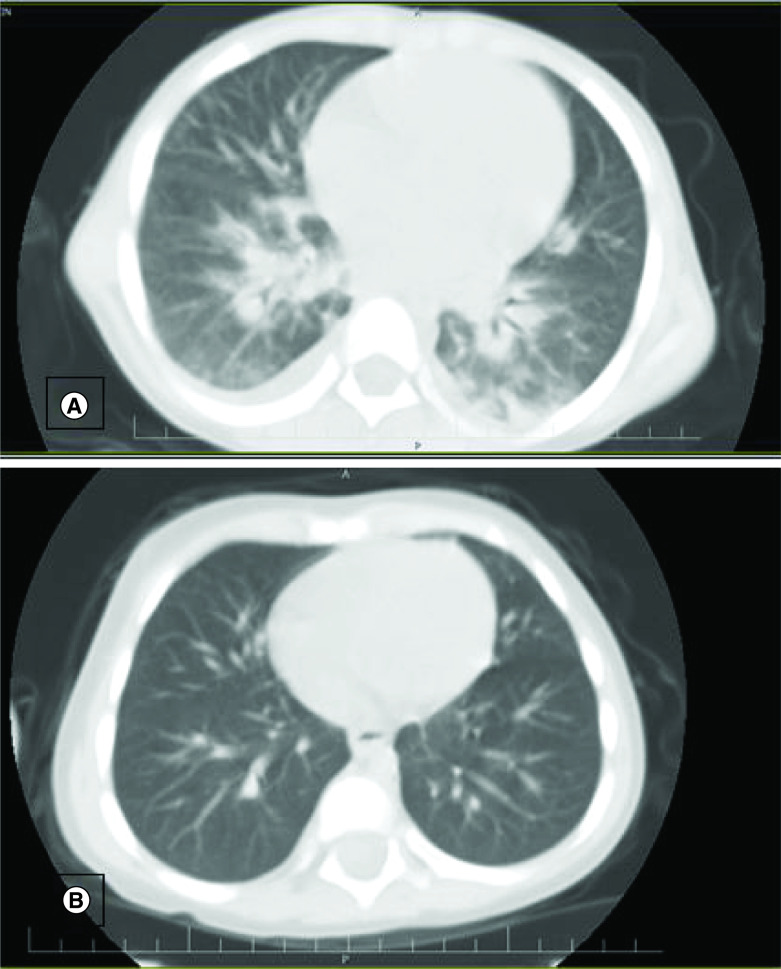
Computed tomography scans of two patients showed bilateral involvement of the lungs with sub-pleural multi lobar interstitial linear opacities and partial pleural effusion. (A) Case 1. (B) Case 2.
